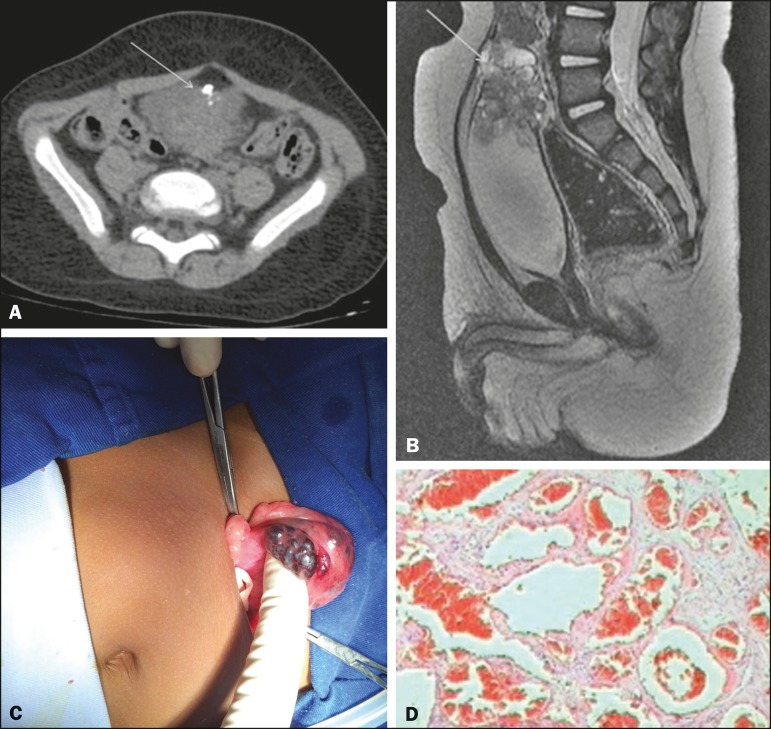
A: Axial computed tomography scan of the abdomen, showing a
